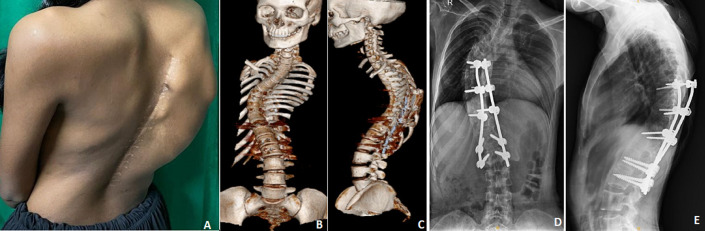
A) clinical photographs of old operated case of adolescent idiopathic scoliosis at dorsal spine with recurrence of deformity; B,C) Computed tomography scan image showing operated case of scoliosis with implant in situ with apex of deformity towards right side and recurrence of deformity start from proximal end of implant; D) anteroposterior view of X-ray image showing operated case of scoliosis with implant in situ with apex of deformity towards right side and concave portion towards left side and recurrence of deformity start from D9 vertebrae; E) lateral view of X-ray image showing operated case of scoliosis with implant in situ with apex of deformity towards right side and concave portion towards left side and recurrence of deformity start from D9 vertebrae
